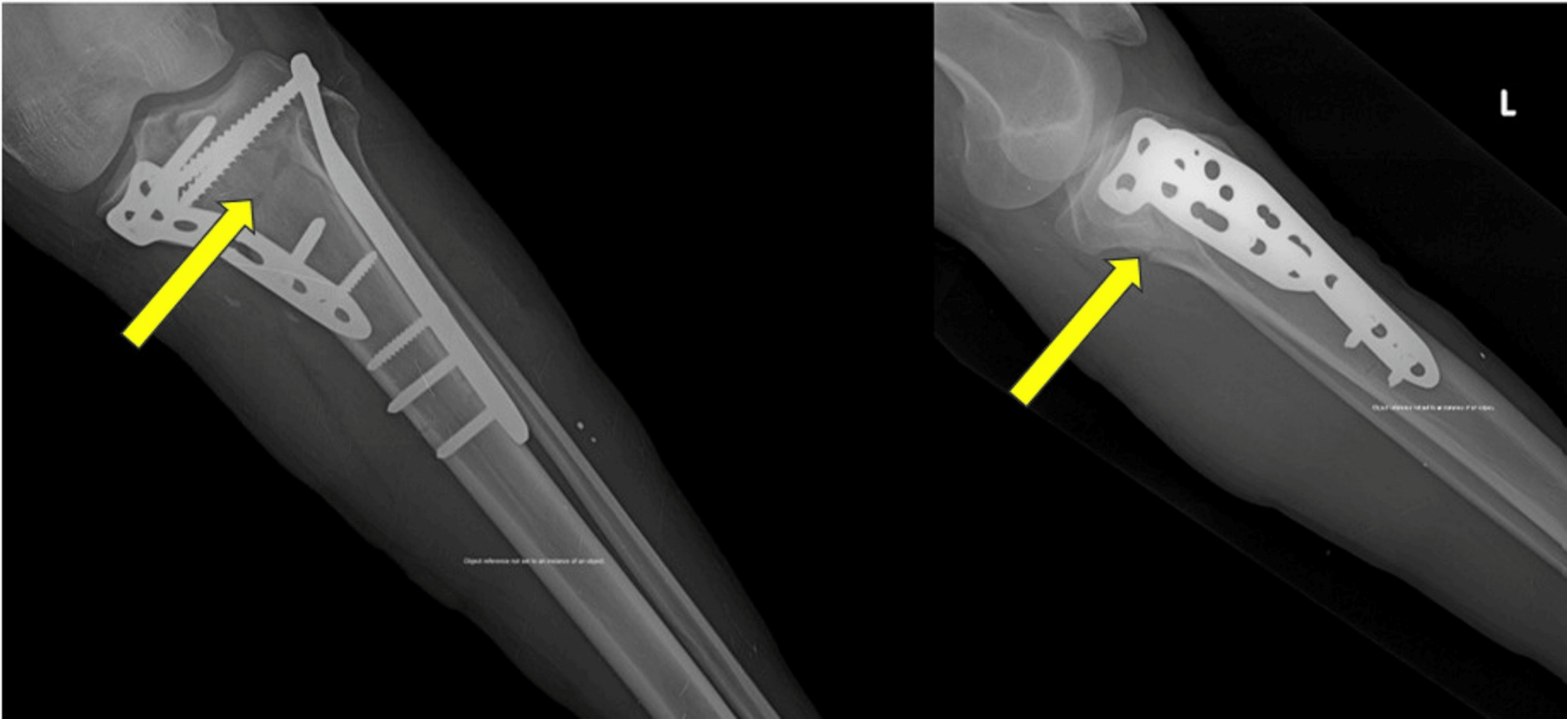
Immediate postoperative radiographs showing dual medial and lateral plate fixation with satisfactory fracture reduction
